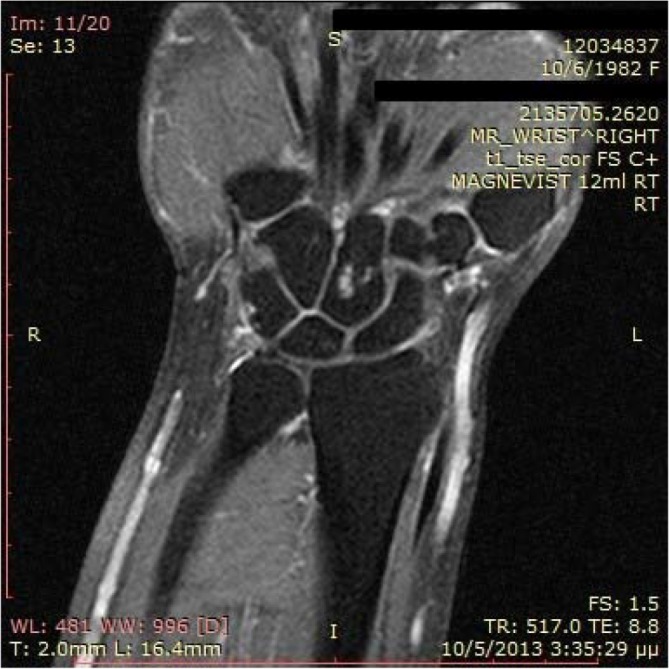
MRI with T1 sequence and use of magnetic contrast media of the patients’ right wrist indicating erosions in multiple sites.
